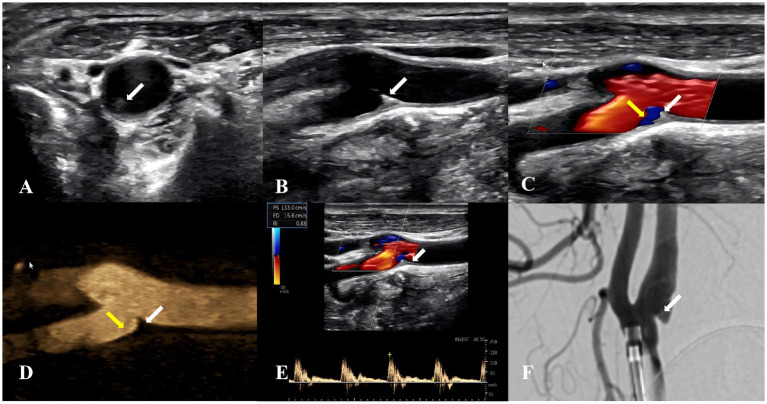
Multimodal ultrasound image of carotid web (white arrows) located in the posterior wall of the right carotid artery at its origin. (A) An isoechoic membrane-like structure is shown in the transverse ultrasound image. (B) A short, thin, and linear bilinear structure in longitudinal ultrasound images. (C) Color Doppler flow imaging revealed swirling flow (yellow arrow) at the base of the carotid web. (D) B-flow imaging delineates the carotid web with high clarity without color Doppler’s overflow artifacts, simultaneously revealing the morphology and localized blood flow disturbances (yellow arrow). (E) Spectral Doppler measurements at the carotid web. (F) Corresponding digital subtraction angiography (DSA) image shows the definitive linear filling defect.
